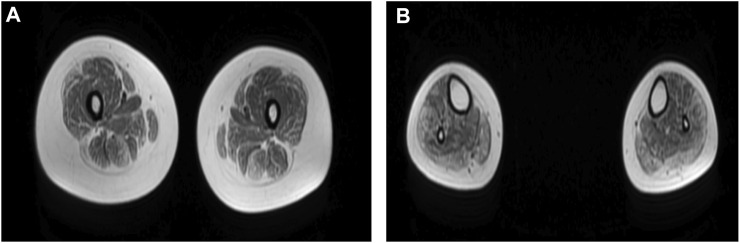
(A,B) Muscle MRI of lower limbs showed fatty infiltration and muscle atrophy of proximal and distal lower limb muscles, with the most prominent involvement in posterior muscles. (A) Thigh level. The biceps femoris (long head), semitendinosus, vastus lateralis, gracilis, and sartorius muscle are mildly to moderately affected. The rectus femoris is the best spared muscle. (B) Calf level. The gastrocnemius (medial head and lateral head), peroneus (fibularis) brevis, and peroneus (fibularis) longus muscle are moderately affected.
